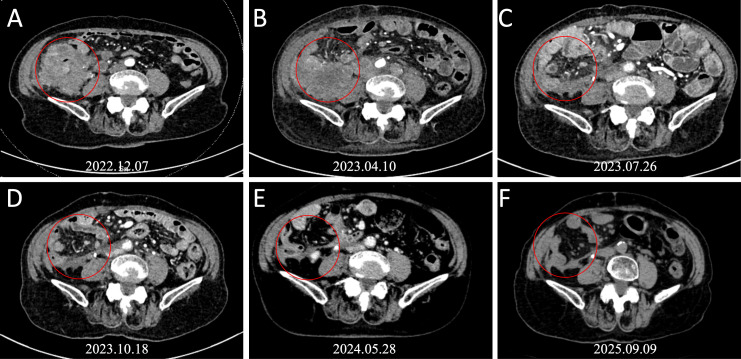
Serial contrast-enhanced abdominal CT scans. (A) Preoperative scan (December 7, 2022) showing thickening of the cecum and ascending colon. (B) Baseline scan at recurrence (April 10, 2023) prior to systemic therapy, showing a right lower quadrant mass and retroperitoneal lymphadenopathy. (C) Follow-up scan (July 26, 2023) after 3 months of combination therapy (pembrolizumab + dabrafenib + trametinib), showing partial response (PR). (D, E) Follow-up scans (May 2024 and others) during pembrolizumab maintenance, showing sustained tumor regression. (F) Most recent follow-up (September 9, 2025) confirming radiographic complete response (CR).
